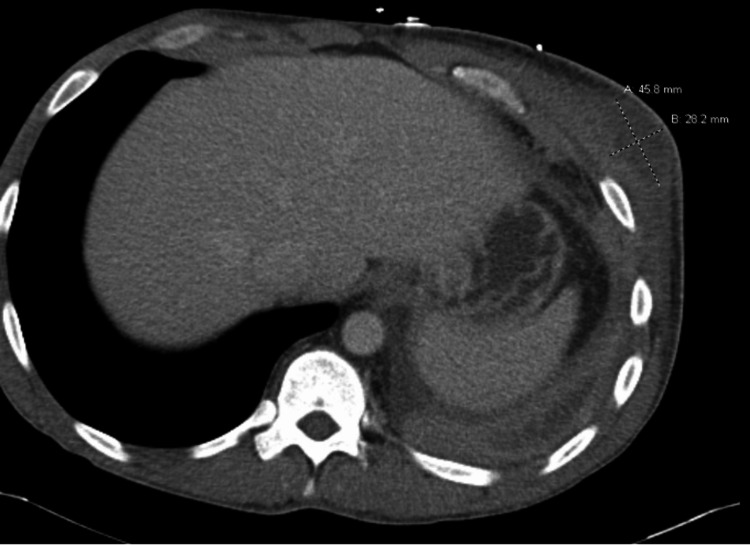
Chest CT on day 2 of admission, suspicious for loculated empyema.
